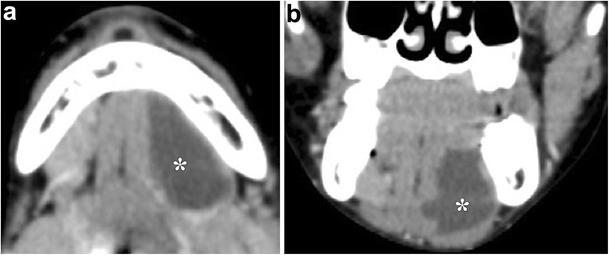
Simple ranula. a Axial and b coronal contrast-enhanced CT images demonstrate a well-circumscribed fluid attenuation lesion within the left sublingual space (*)
